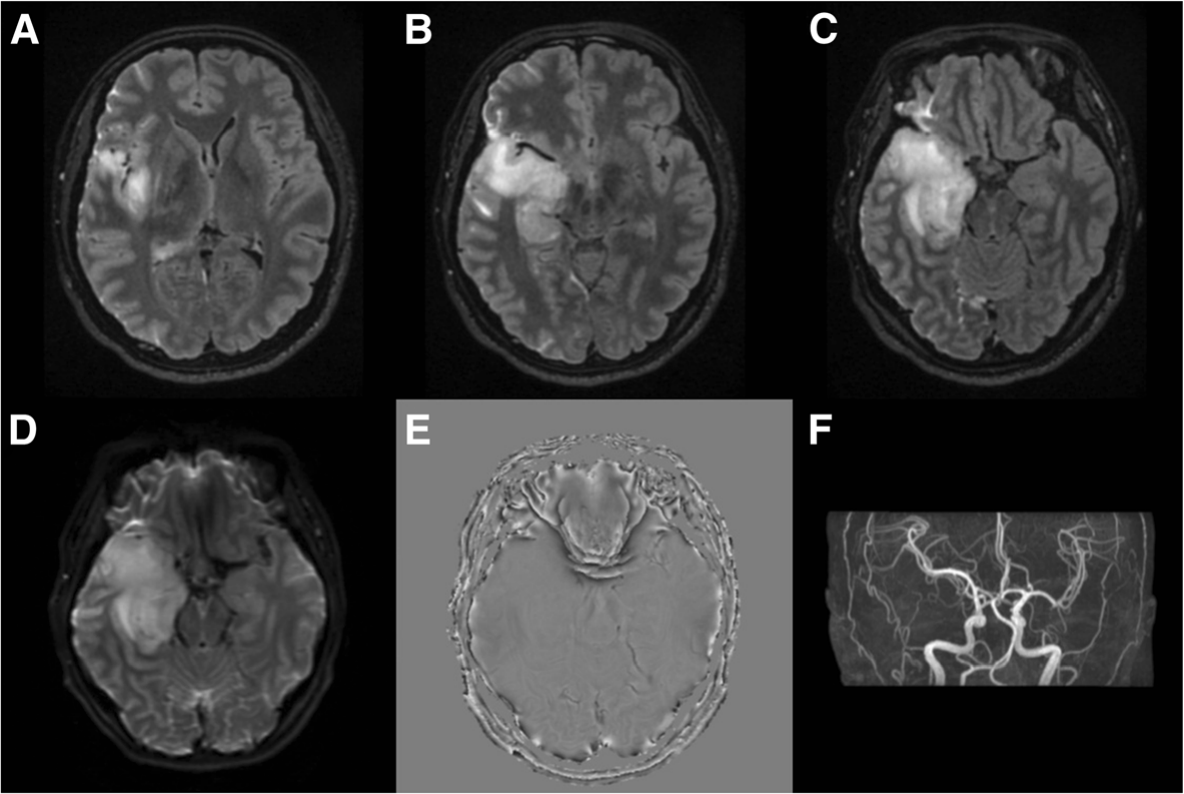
Initial brain MRI (2017.12.15). T2 weighted image shows diffuse swelling and high signal intensity changes of the right (a) insula (b) hippocampus (c) temporal lobe. d Diffusion weighted image (DWI) shows diffusion restriction of the right temporal lobe lesion (e) Susceptibility weighted angiography (SWAN) shows no evidence of hemorrhage (f) Brain MR Angiography shows no steno-occlusive lesion or aneurysm in intracranial vessels
